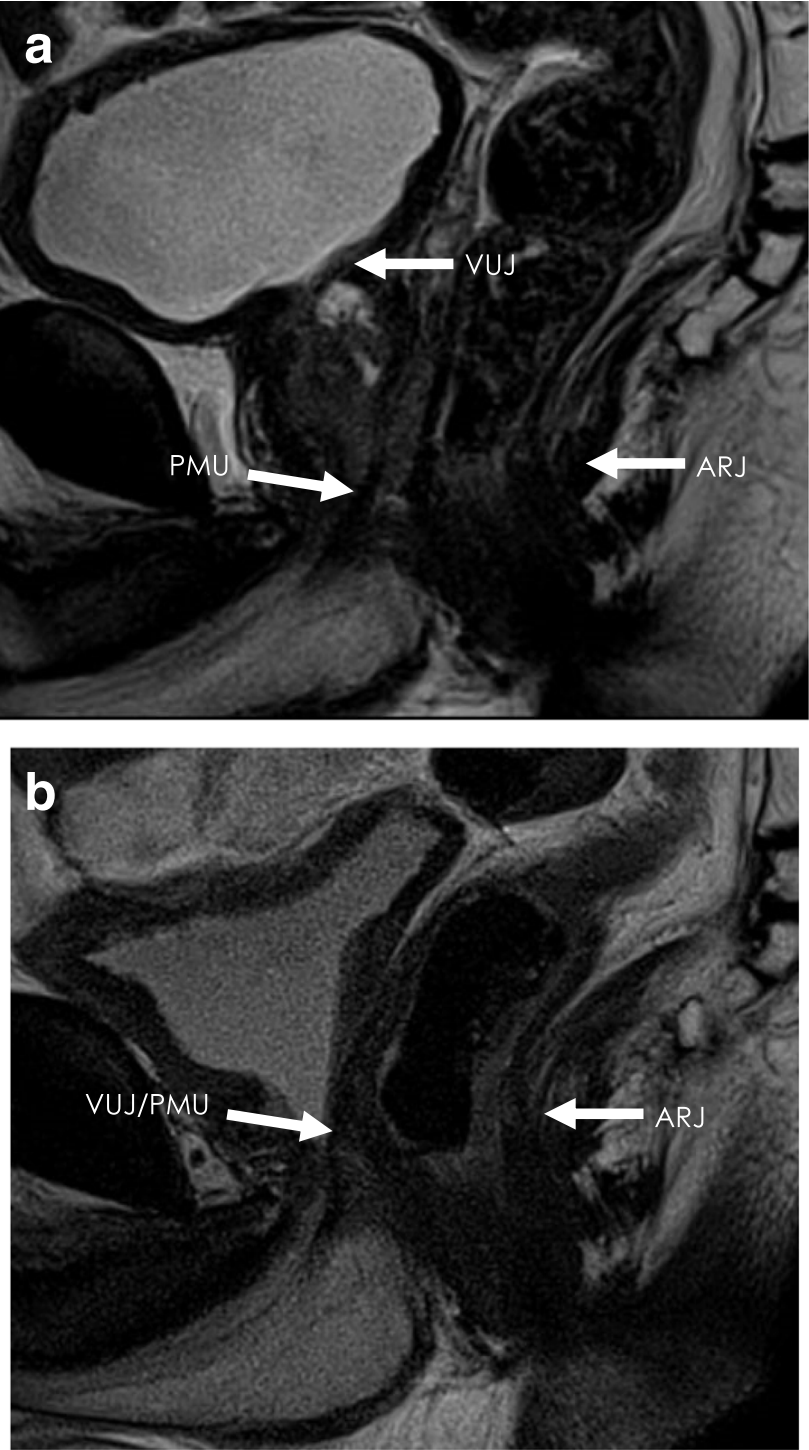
The a) preoperative and b) postoperative T2W midsagittal MRI images showing the VUJ, PMU and ARJ anatomical landmarks
